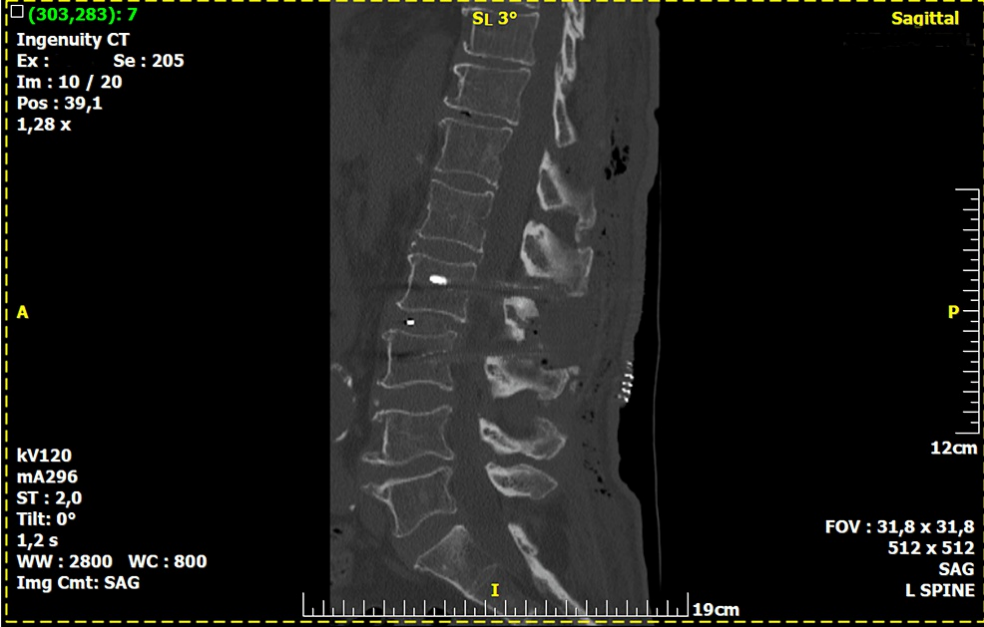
Postoperative CT scan of the lumbar spine Saggital view of CT scan showing satisfactory spinal alignment
